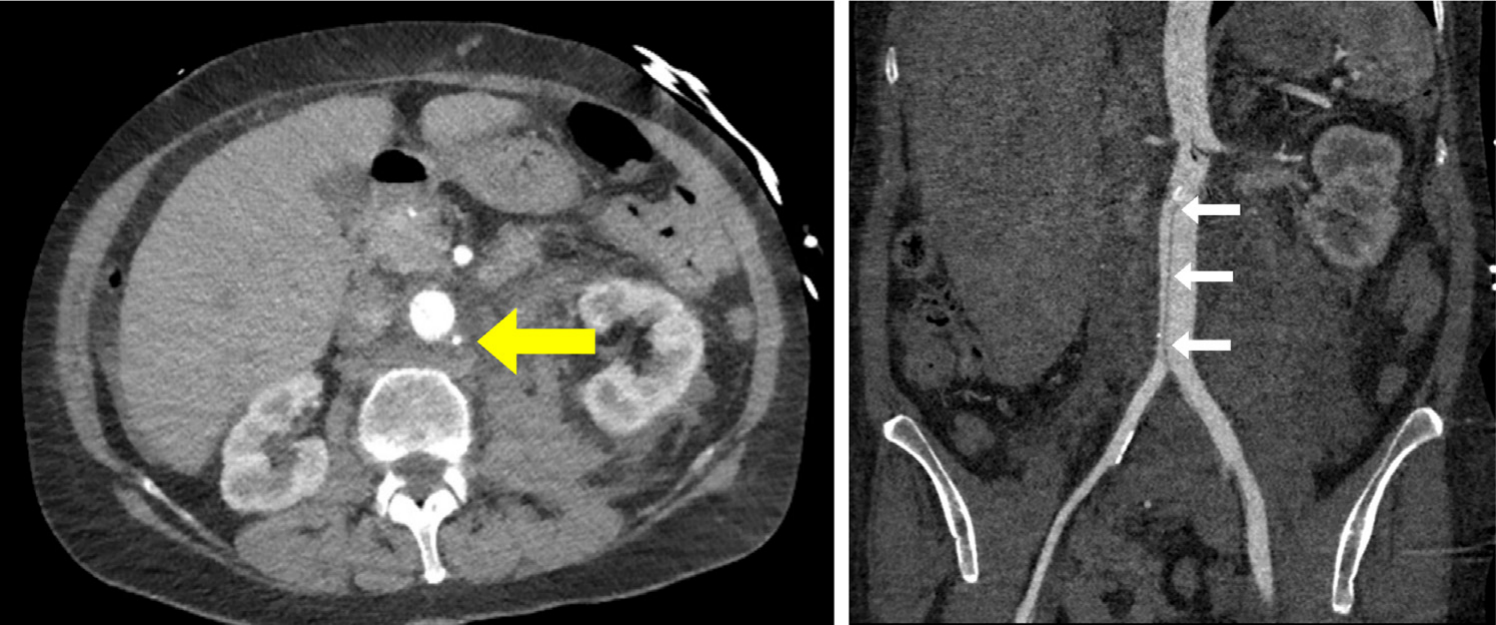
Axial computed tomography (CT) demonstrating the inferior aspect of the intra-aortic balloon pump (IABP) terminating external to the aorta (*yellow arrow*) and coronal multiplanar reconstruction CT demonstrating aortic dissection extending from the infrarenal aorta to the left external iliac arteries (*white arrows*).
